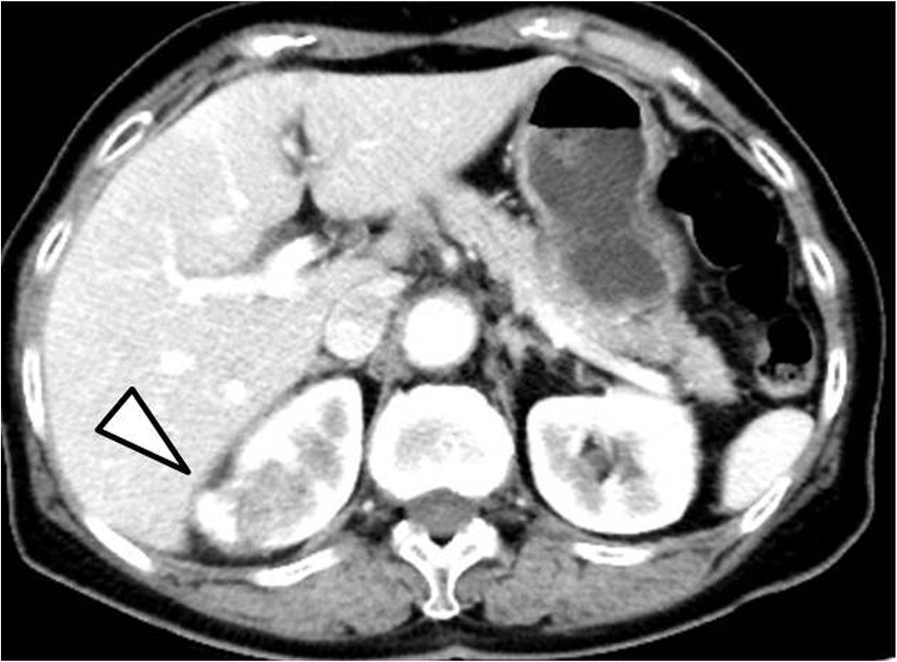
Enhanced abdominal computed tomography (CT). Abdominal CT revealed a hypovascular tumor with massive calcification, measuring 2.5 × 2.3 cm, in the upper pole of the right kidney (arrow).
